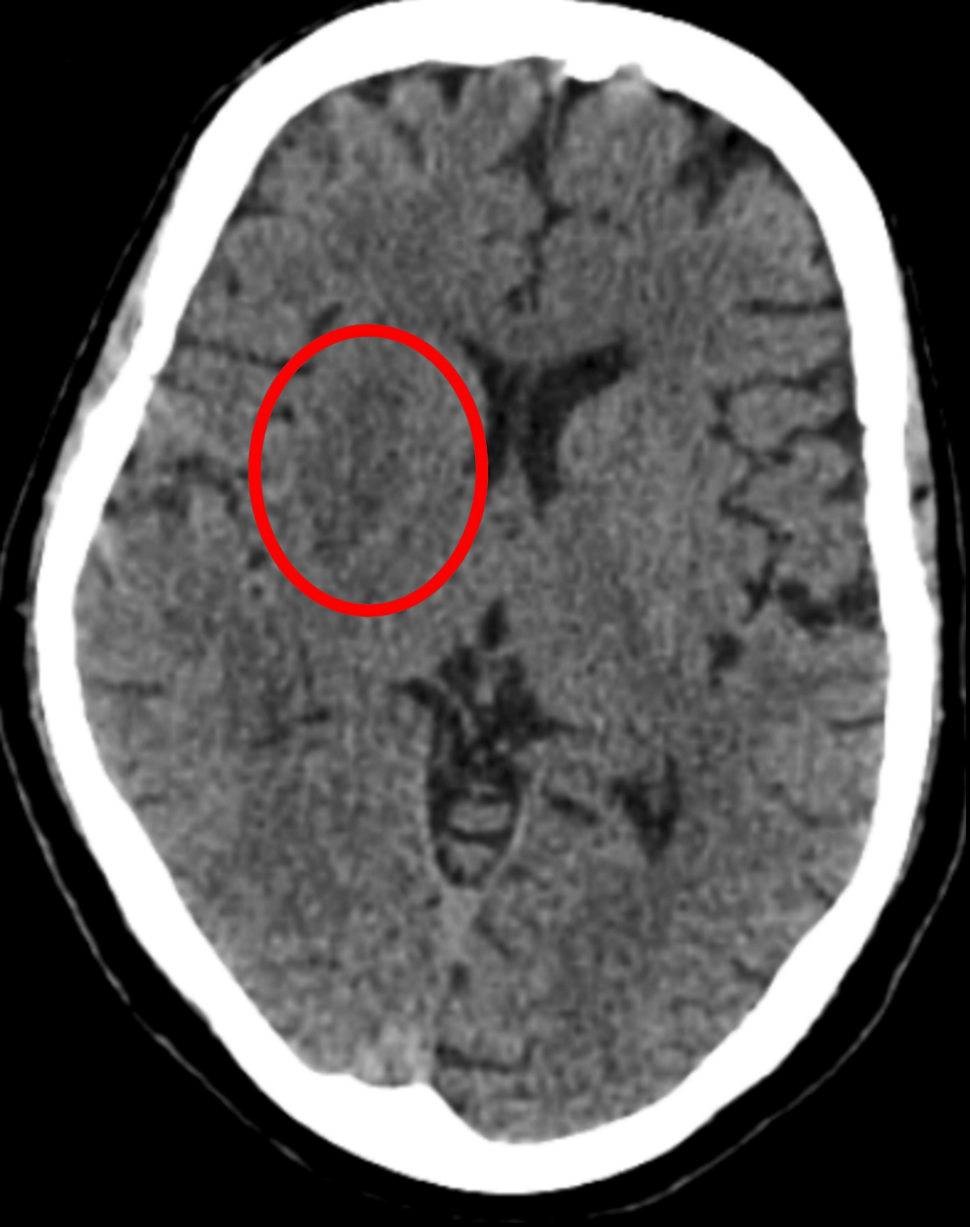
CT brain of a 58 years old, who presented with left side weakness The image shows a hypodense area involving the right capsuloganglionic region, suggestive of acute nonhemorrhagic infarct, which was missed in the provisional report. Original CT brain image of a patient clinically suspected of acute infarct
